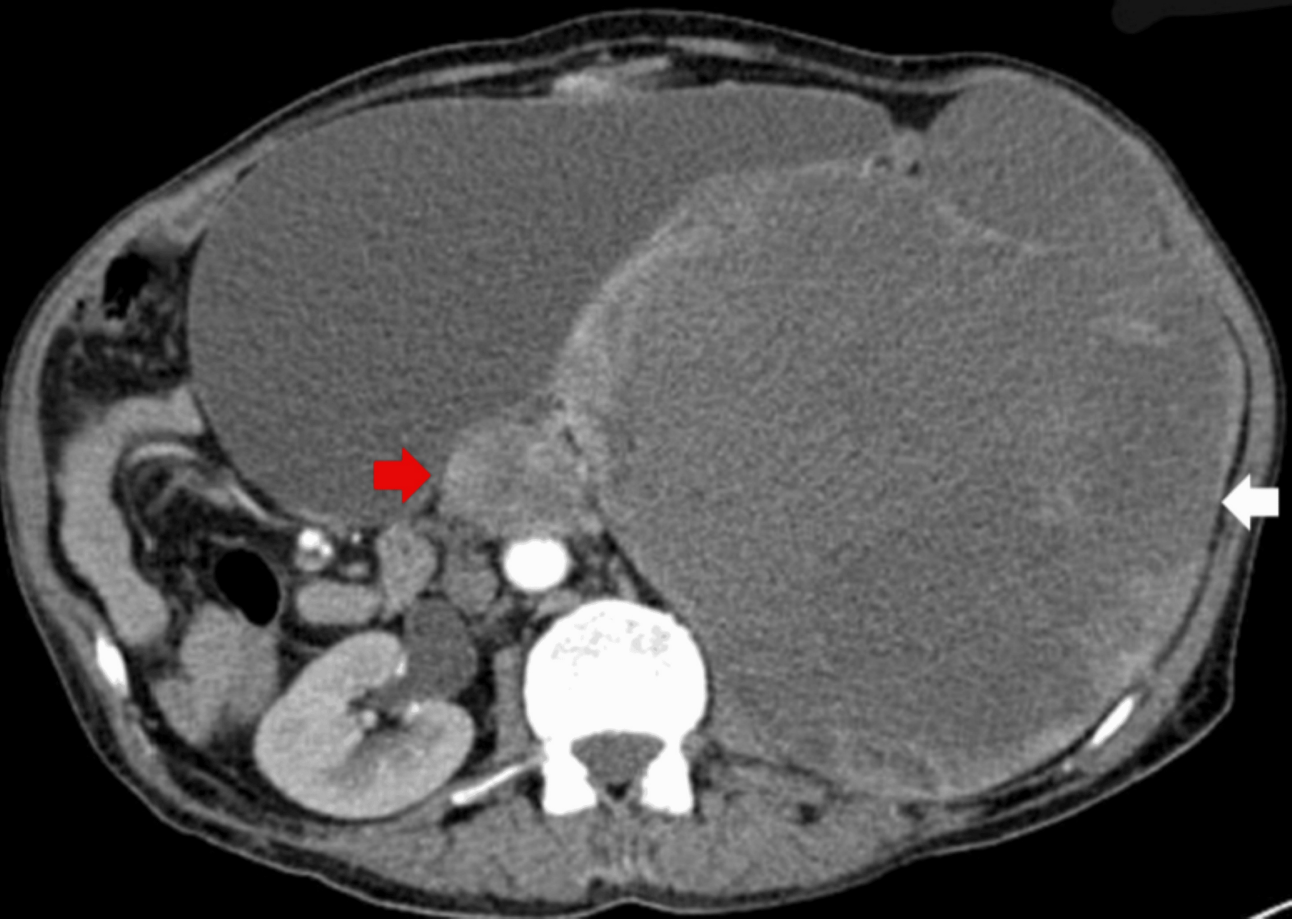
Contrast-enhanced CT (Axial view) at the level of the upper abdomen showing heterogeneous left renal mass. The mass demonstrates a peripherally enhancing soft tissue component with a large central area of necrosis (white arrow). Rounded enhancing soft tissue lesion with central necrosis, measuring 4.5 × 4 cm, is seen anterior to the aorta below the origin of the renal arteries, exhibiting similar characteristics to the primary mass (red arrow)
